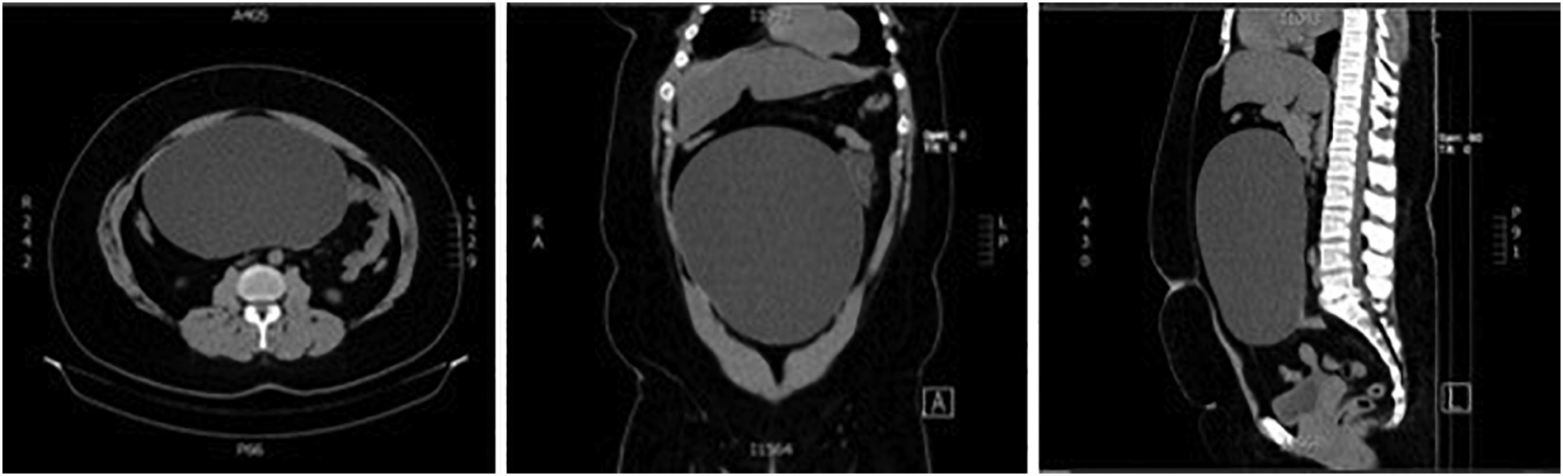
CT scan showing a giant central cyst.
